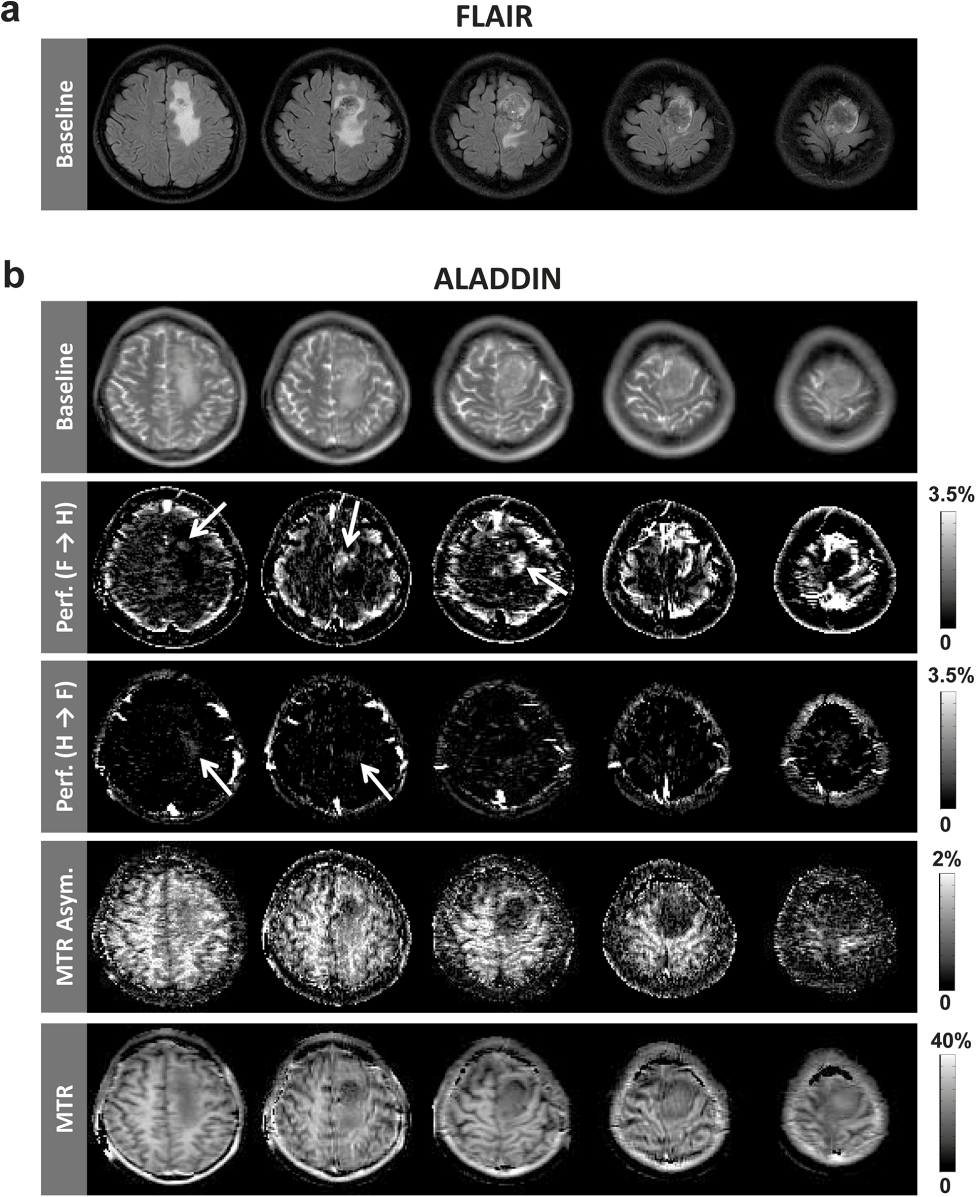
Clinical demonstration of simultaneous mapping of cerebral blood flow, MTR_Asym_, and MTR with ALADDIN. a: FLAIR images. The total scan time was ~2.4 min. b: ALADDIN images acquired from a brain tumor patient. The arrows indicate heterogeneous blood flow directionality in the tumor region. The data were acquired with centric PE order and scan time was ~5.7 min for ALADDIN acquisition and ~1.7 min for acquisition of MT-free images (total ~7.4 min).
